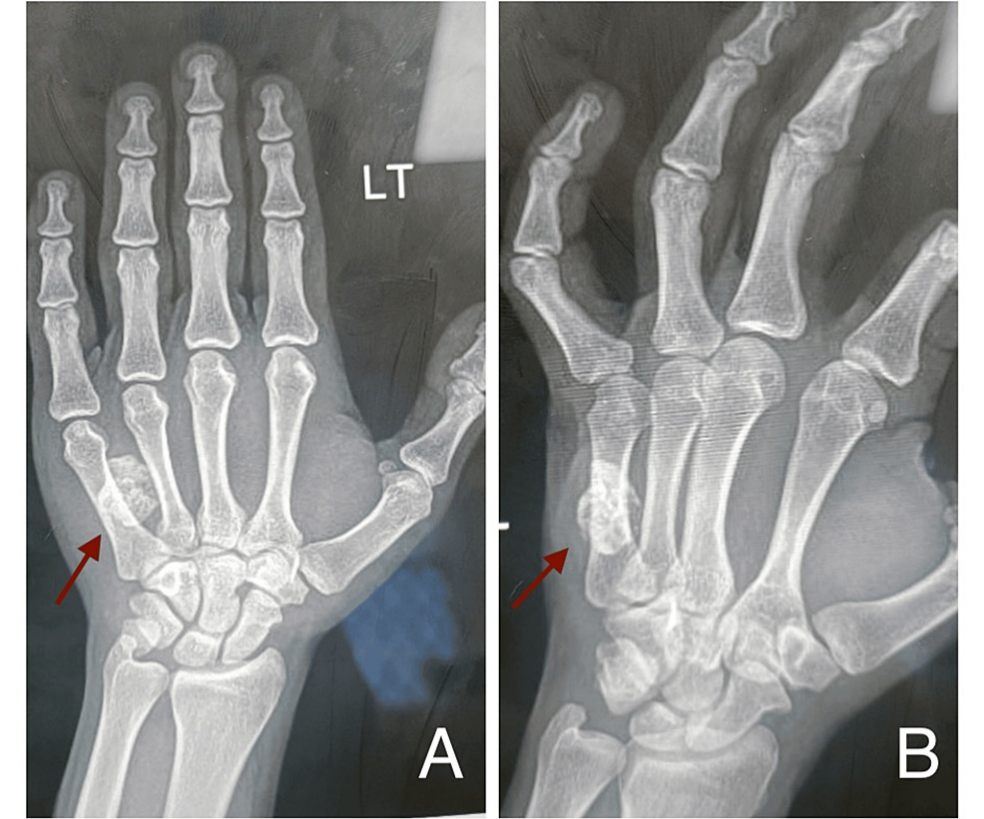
Image showing the anterior-posterior (Figure A) and oblique (Figure B) X-ray views of the left hand with the calcified lesion over the shaft of the fifth metacarpal bone extending to the fourth inter-metacarpal space.
